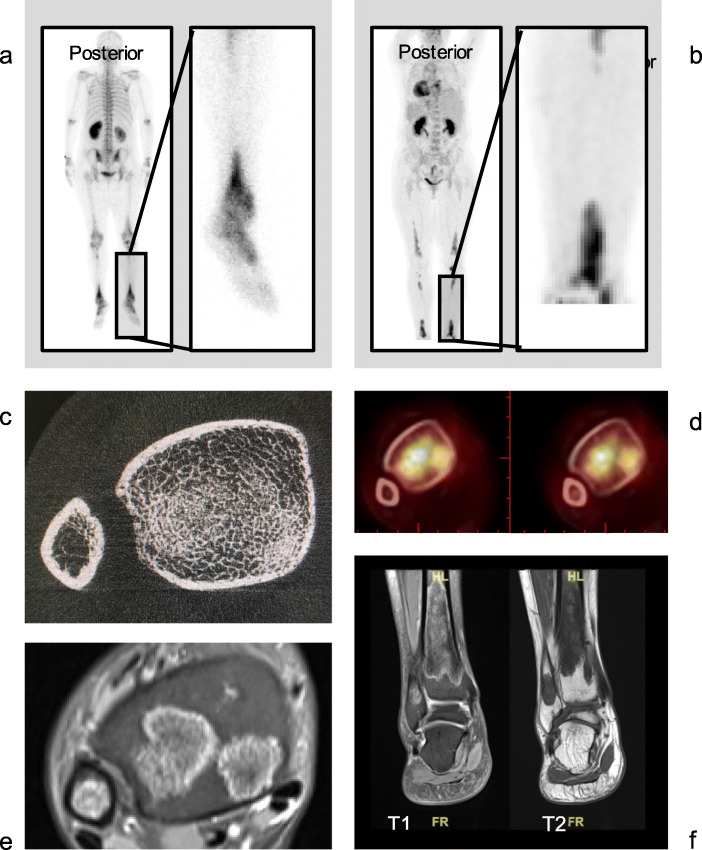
Images of bone scintigraphy, positron emission tomography-computed tomography (PET-CT), HR-pQCT and Magnetic Resonance Imaging. (MRI) in one representative treatment-naïve ECD patients (LJ008). a Bone scintigraphy showed bilateral symmetric uptake at the distal tibial region. b PET-CT showed abnormal uptake at the same tibial region. c HR-pQCT revealed localized structural alteration of trabeculae network in right tibia. d Cross-sectional view of PET-CT showed increase of uptake in tibia, where bone lesions existed, reaching a SUV maximum of 5. e and f MRI of affected left ankle showed localized heterogeneous low T1- and T2-signal, resembling that of cortex
